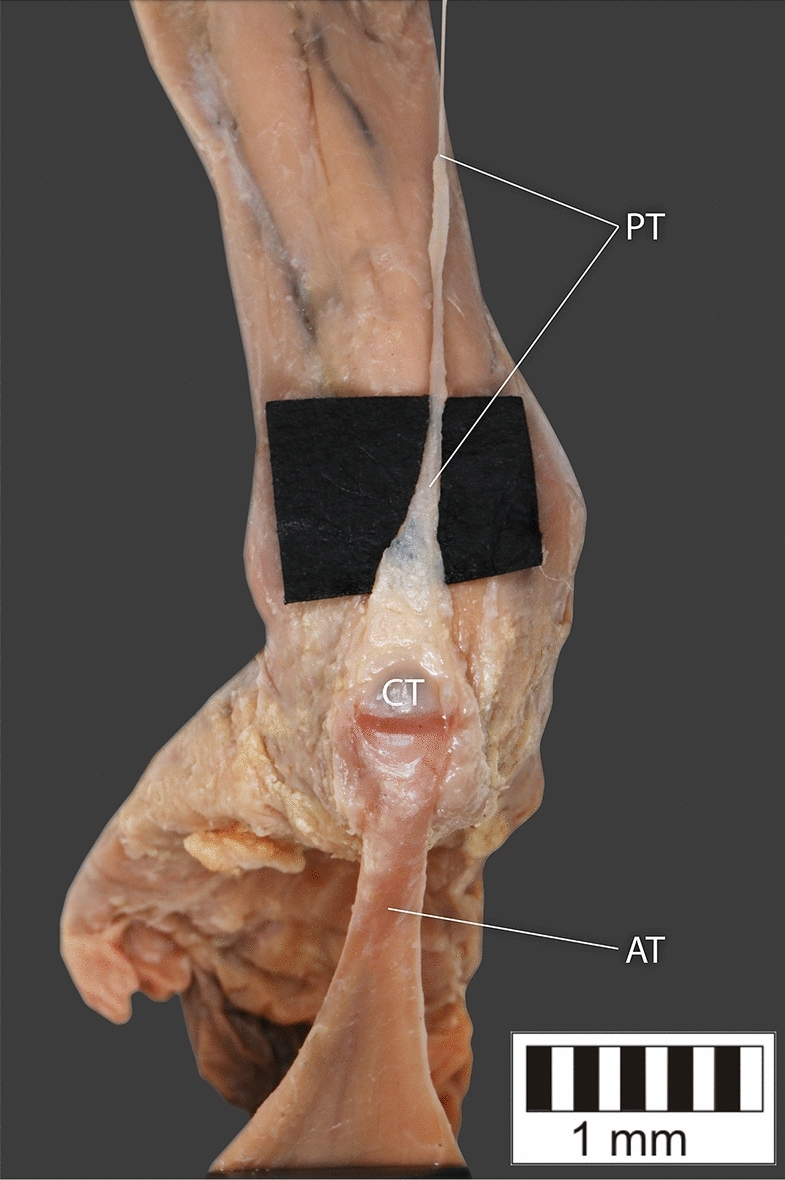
Type III of plantaris tendon. *CT* calcaneal tuberostity, *AT* Achilles tendon, *PT* plantaris tendon.
